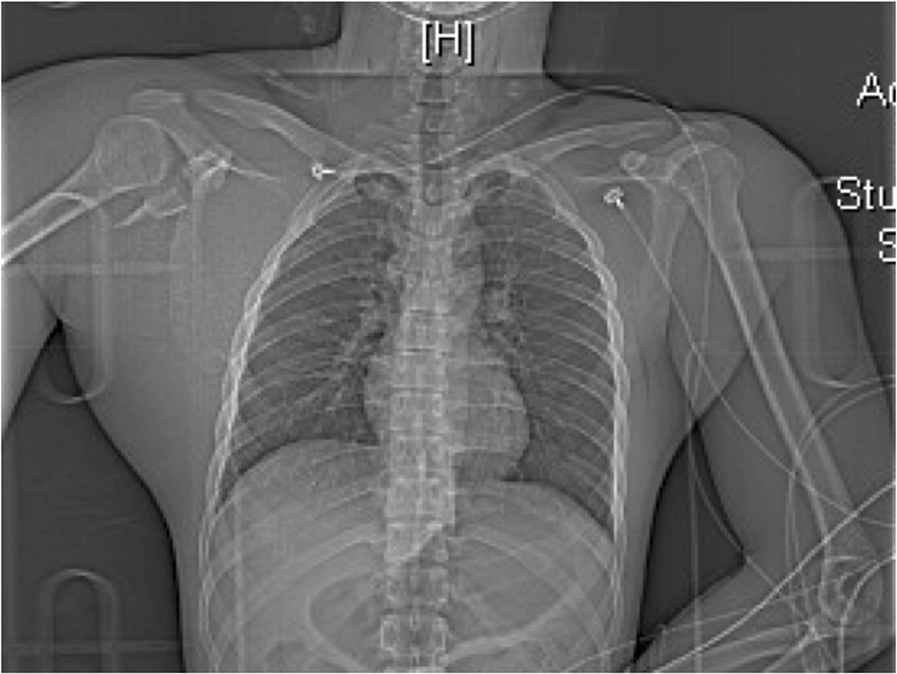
CT scout film. CT showing a fracture through the right scapular neck and marked soft tissue swelling of the right shoulder and chest wall
